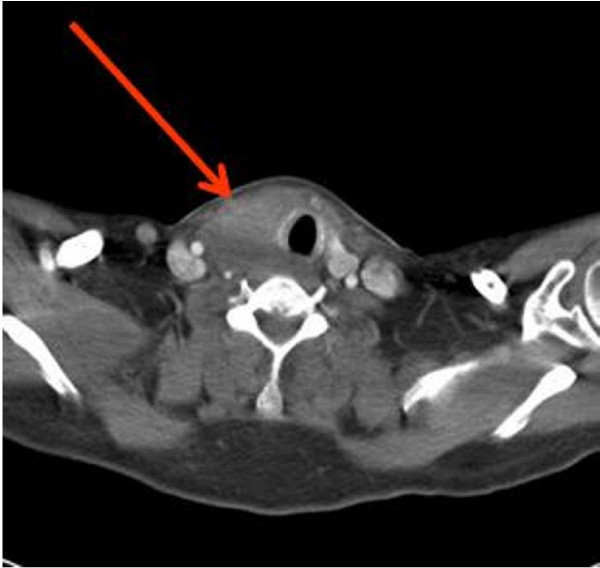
Computed tomography (CT) scan of the neck and the thoracic outlet. This shows a large thyroid mass predominantly affecting the right lobe of the thyroid gland. The trachea has been pushed over to the left.
